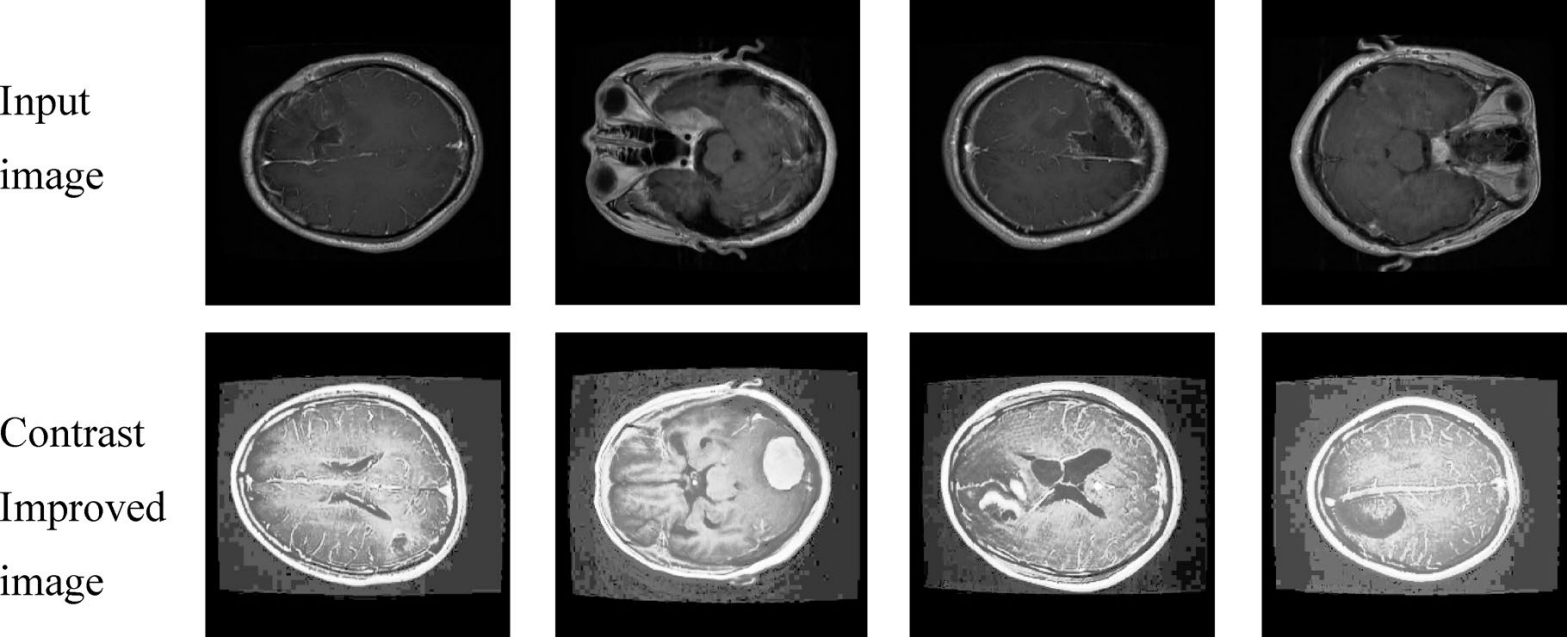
Enhanced brain MRI images using histogram equalization for improved contrast.
